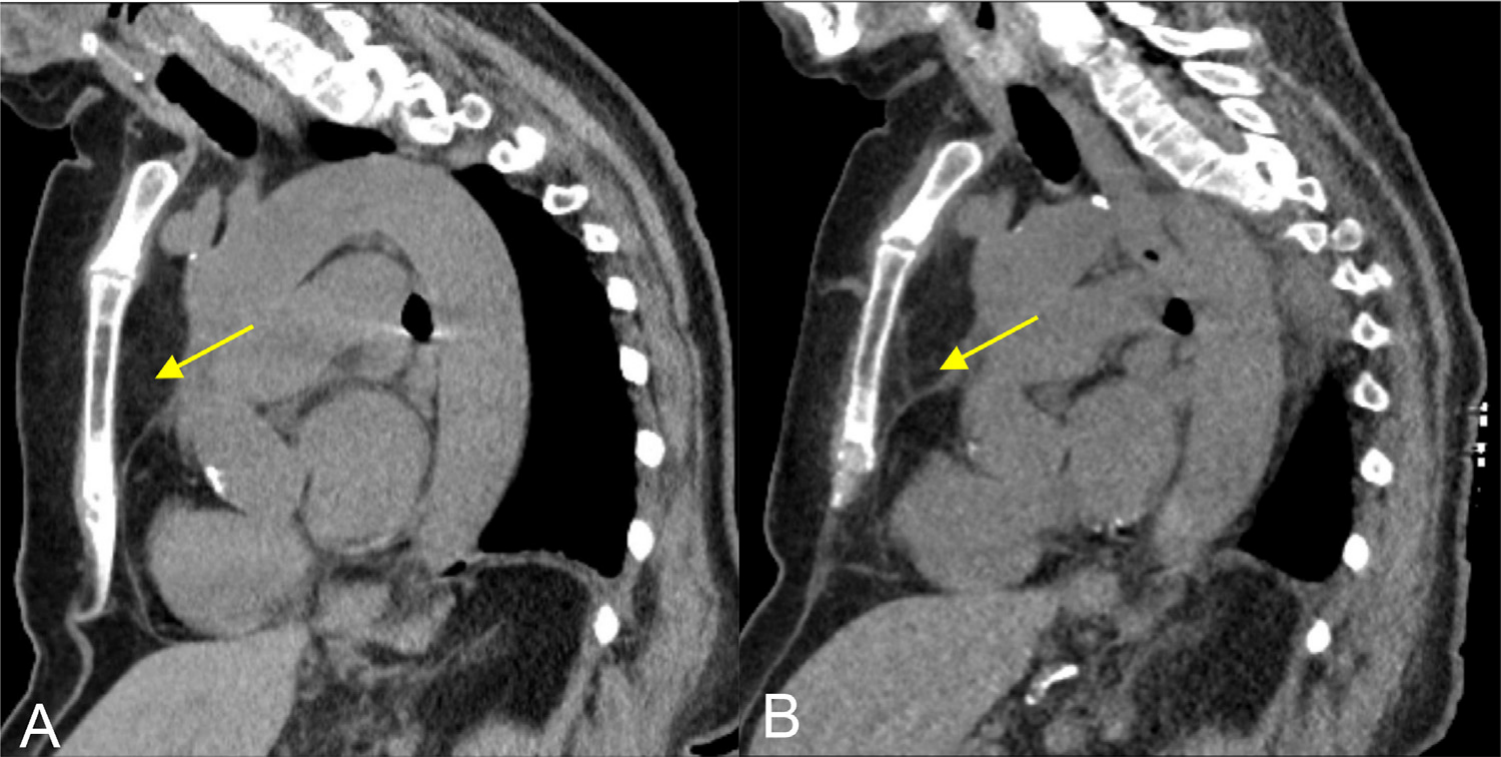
Most recent CT chest (left) compared to prior CT Chest 2 months prior (right). (A) Retrosternal soft tissue stranding (yellow arrow) showed improvement in the most recent follow-up study. T5-T9 endplate sclerosis was unchanged (not shown). (B) Prior CT Chest with retrosternal soft tissue inflammation (yellow arrow).
